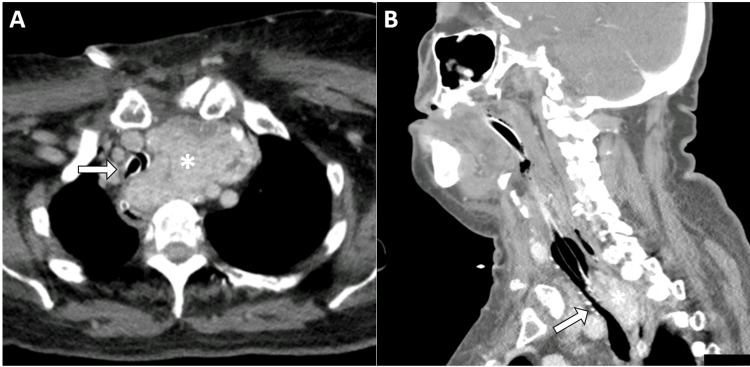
Postoperative CT scan performed after initial surgery. Axial (A) and saggital (B) images showed residual substernal left goiter (asterisks), measuring 8.3 x 5.1 x 5.9 cm (transverse x anteroposterior x longitudinal), causing significant tracheal compression (white arrows).
